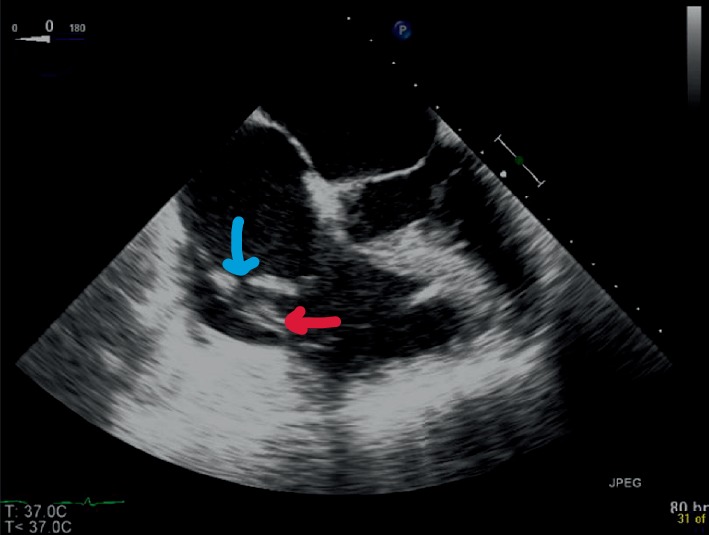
Transesophageal echocardiogram at the midesophageal four chamber view showing rupture of the papillary muscle (red arrow) resulting in protrusion of the tricuspid valve and subvalvular apparatus into the right ventricle, and detached anterior leaflet from the annulus (blue arrow). Significant dilatation of right atrium, with increased pressure as demonstrated by the bowing of the interatrial septum into the left atrium.
